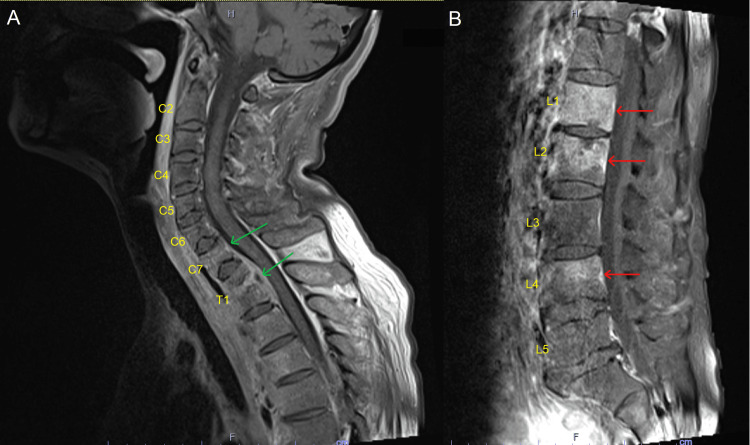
T1-weighted magnetic resonance imaging (MRI) of the spine (A) A stable destructive process at the C7-T1 vertebral levels (green arrows). (B) A stable destructive process at the L1-L4 vertebral levels (red arrows).
